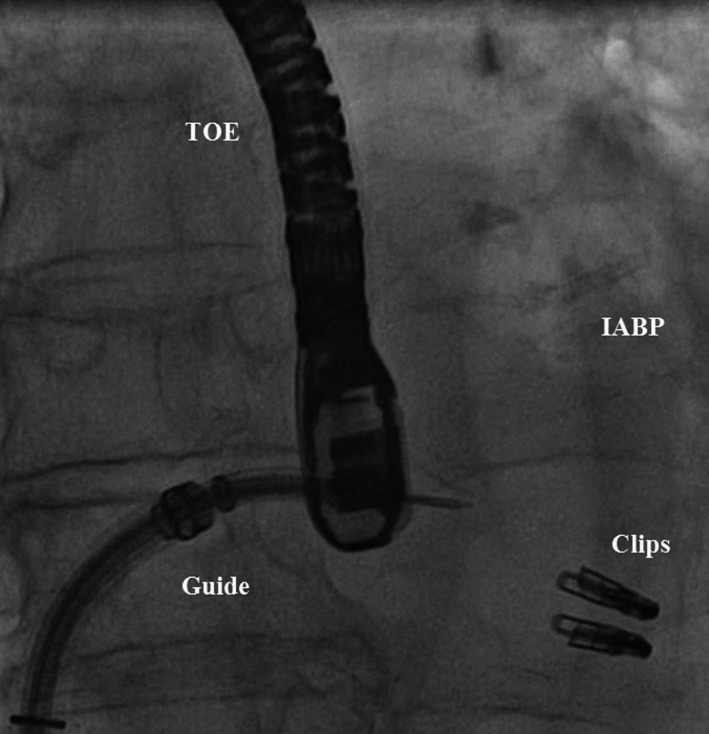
Intraprocedural view: TOE, transesophageal echocardiography; IABP, intra‐aortic balloon pump.
